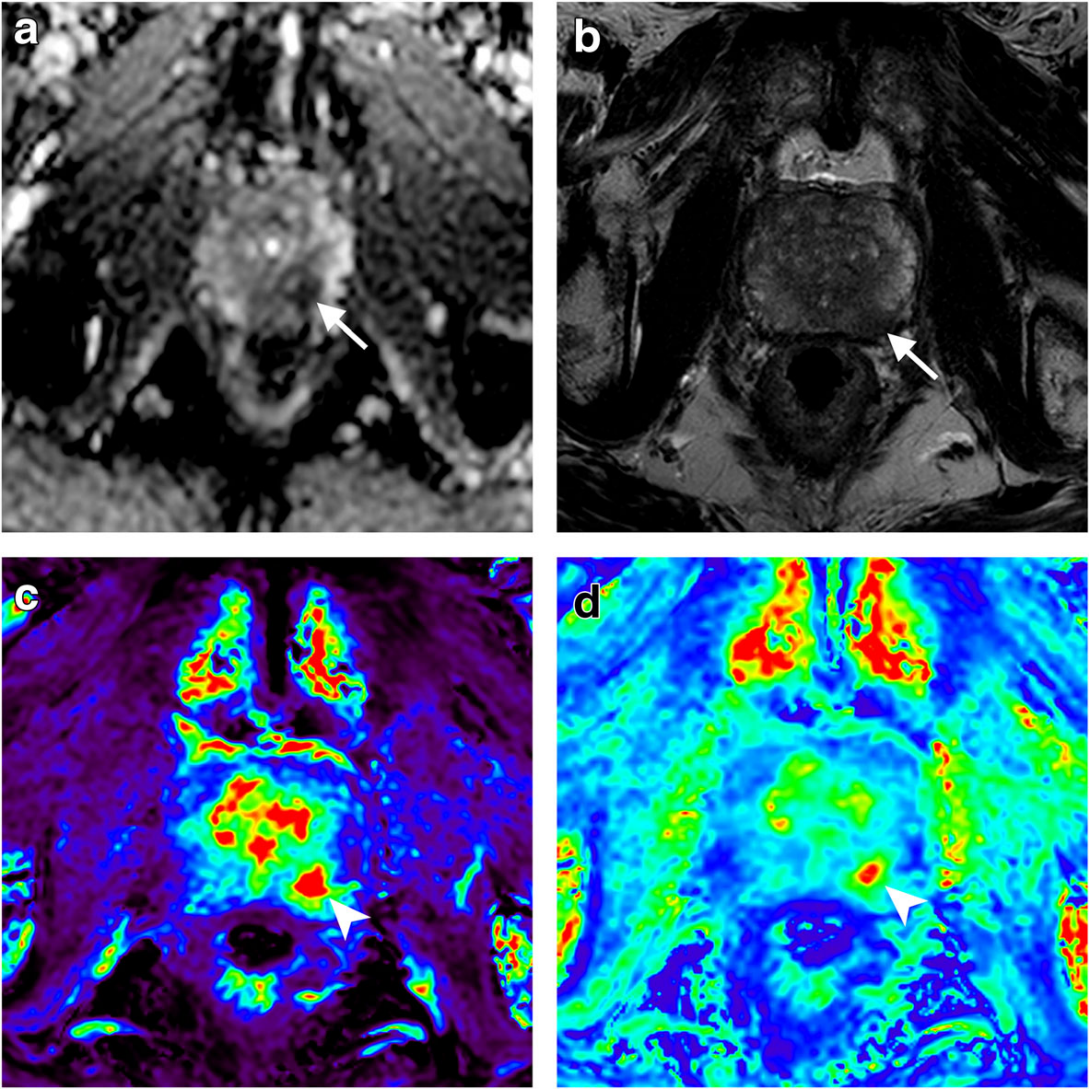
Quantitative DCE imaging in a 71-year-old man undergoing pre-biopsy mpMRI for a left mid PZ cancer (Gleason score 3 + 3 on pathology after radical prostatectomy), showing restricted diffusion on the ADC map (a) and hypointensity on T2-weighted imaging (b) (arrows). DCE parametric maps obtained using the extended Tofts model showed markedly increased *K*^trans^ (arrowhead in c) and *K*_ep_ (arrowhead in d) compared to the surrounding PZ (see Table 4 for details)
